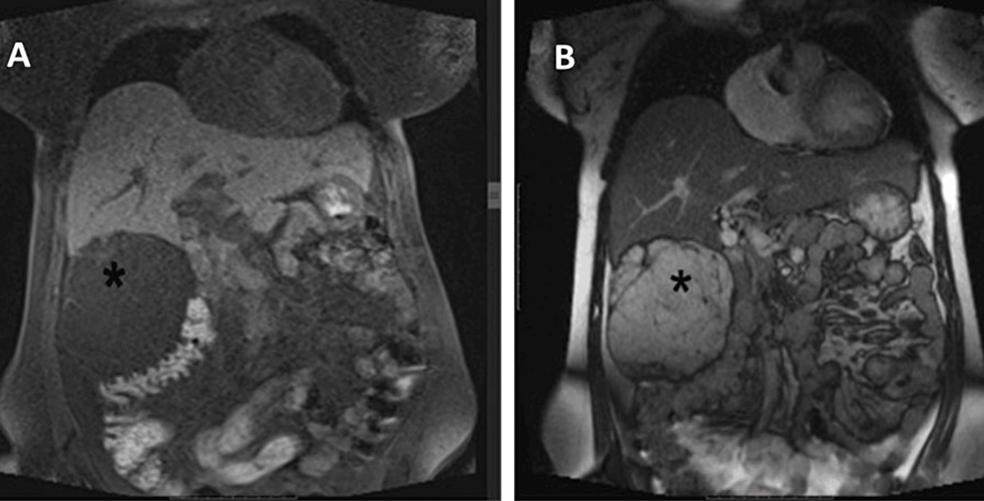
(A) Coronal T1 fat-suppressed and (B) coronal T2WI demonstrate the liver as the organ of origin with mass (marked with an asterisk) showing suppression of signals on fat, thin internal septae and soft tissue. T2WI: T2-weighted image
